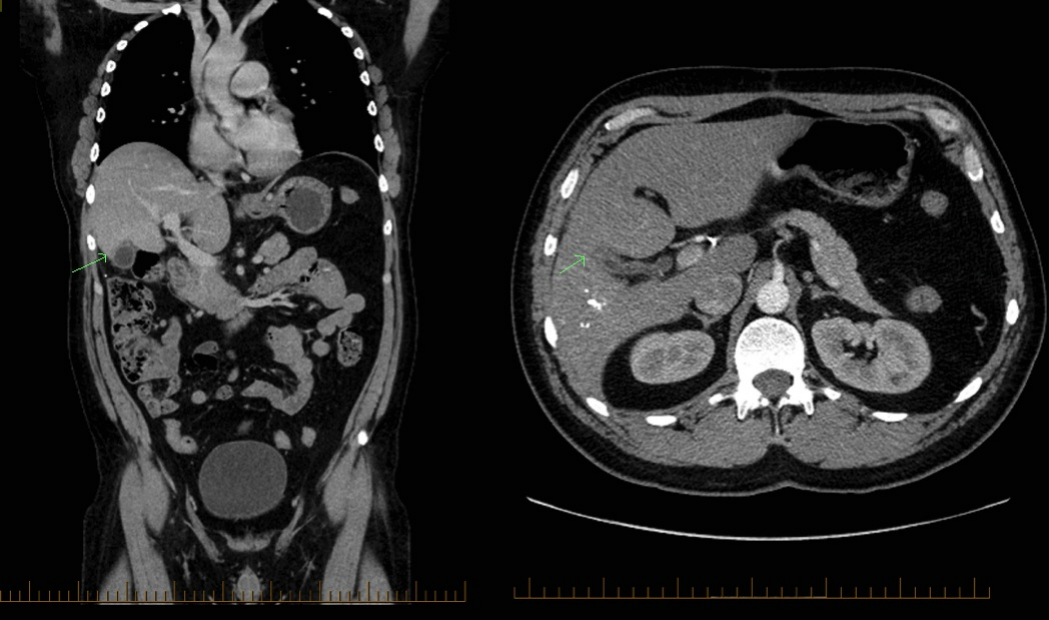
Follow-up CT of the abdomen and pelvis with contrast Coronal (left) and transverse (right) view of the CT of the abdomen and pelvis with contrast showing no sizable gallbladder mass halfway through the fourth cycle of gem/cis chemotherapy.
